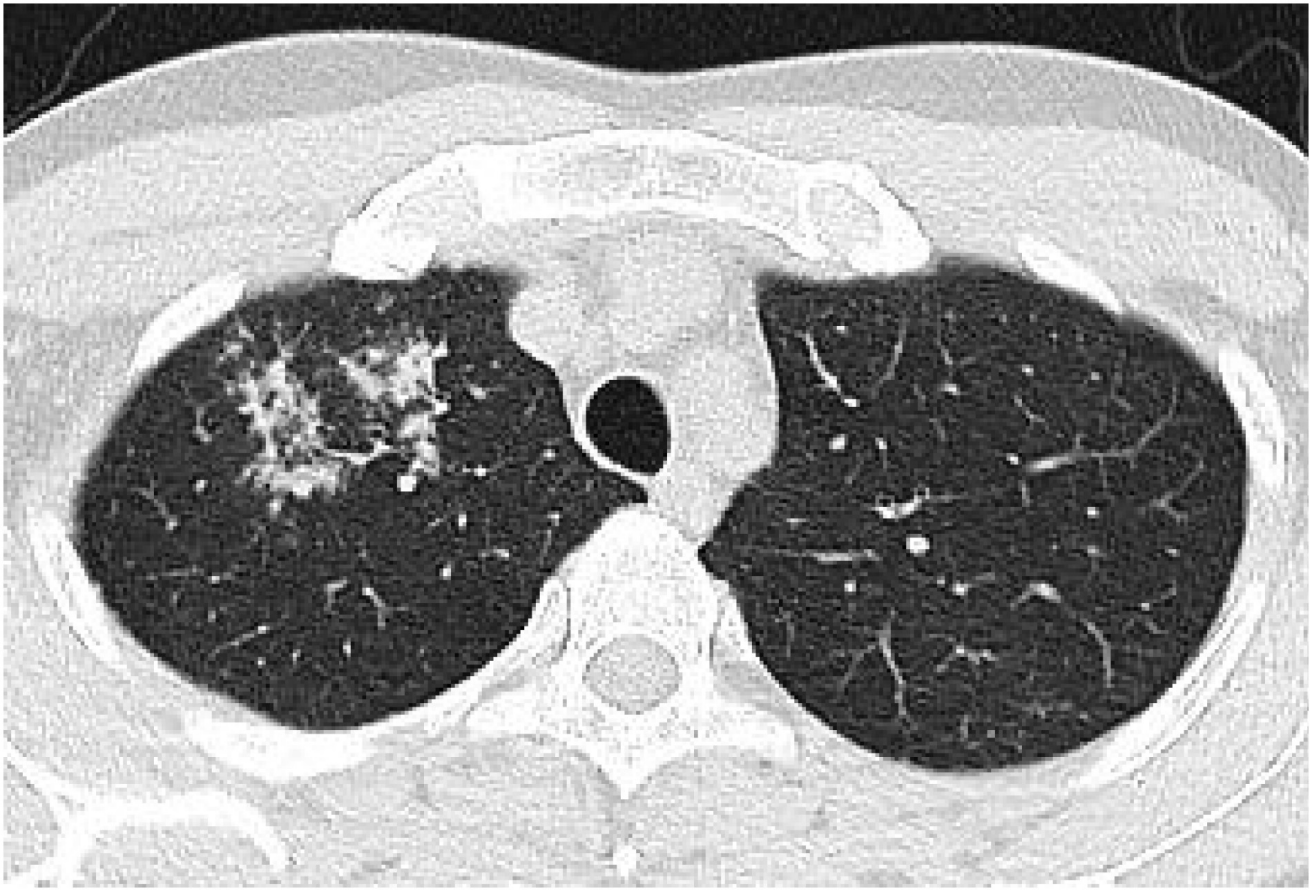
A 30-year-old man with pulmonary TB with negative sputum MTB/RIF. Chest CT shows centrilobular nodules confined to the apical segment of the right upper lobe with peripheral location. Sputum Xpert MTB/RIF was negative, but he was confirmed as active pulmonary TB after positive Xpert MTB/RIF assay on bronchoalveolar lavage.
